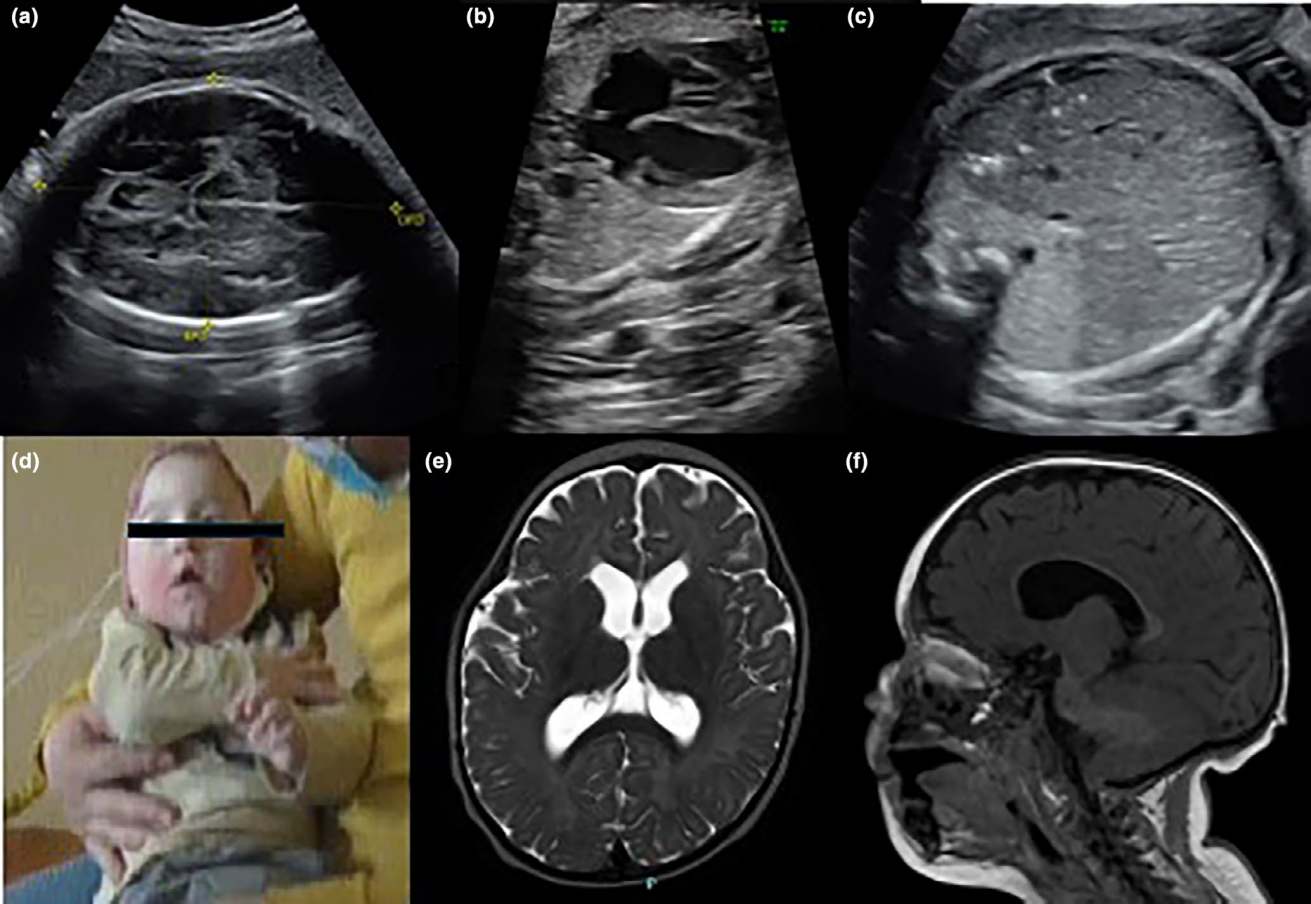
Prenatal unclear scenario in a case with a postnatal diagnosis of Arboleda–Tham syndrome, a recently described genetic disease due to *KAT6A* pathogenic variant and associated with intellectual disability. (a–c) Prenatal ultrasound (31 weeks of gestation) showed an abnormal cranial shape with dolichocephaly (a), abnormal cardiac axis (b), and liver calcifications (c). (d) The patient at 1 year of life showing microcephaly and severe global delay. (e, f) Cerebral magnetic resonance image (10 months of age) showing supratentorial ventriculomegaly, reduced white matter, thin corpus callosum, and tonsillar descent (Arnold–Chiari type 1 malformation).
